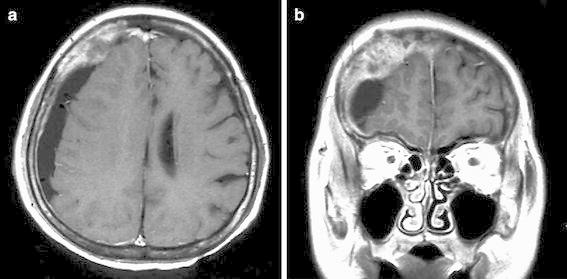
T1-weighted magnetic resonance with gadolinium (a horizontal section, b coronal section), revealing subdural fluid collection on the right side, with a tumor growing into the subdural space and invading the skull bone and dura mater. However, no other metastatic lesions were observed in the brain and meninges
